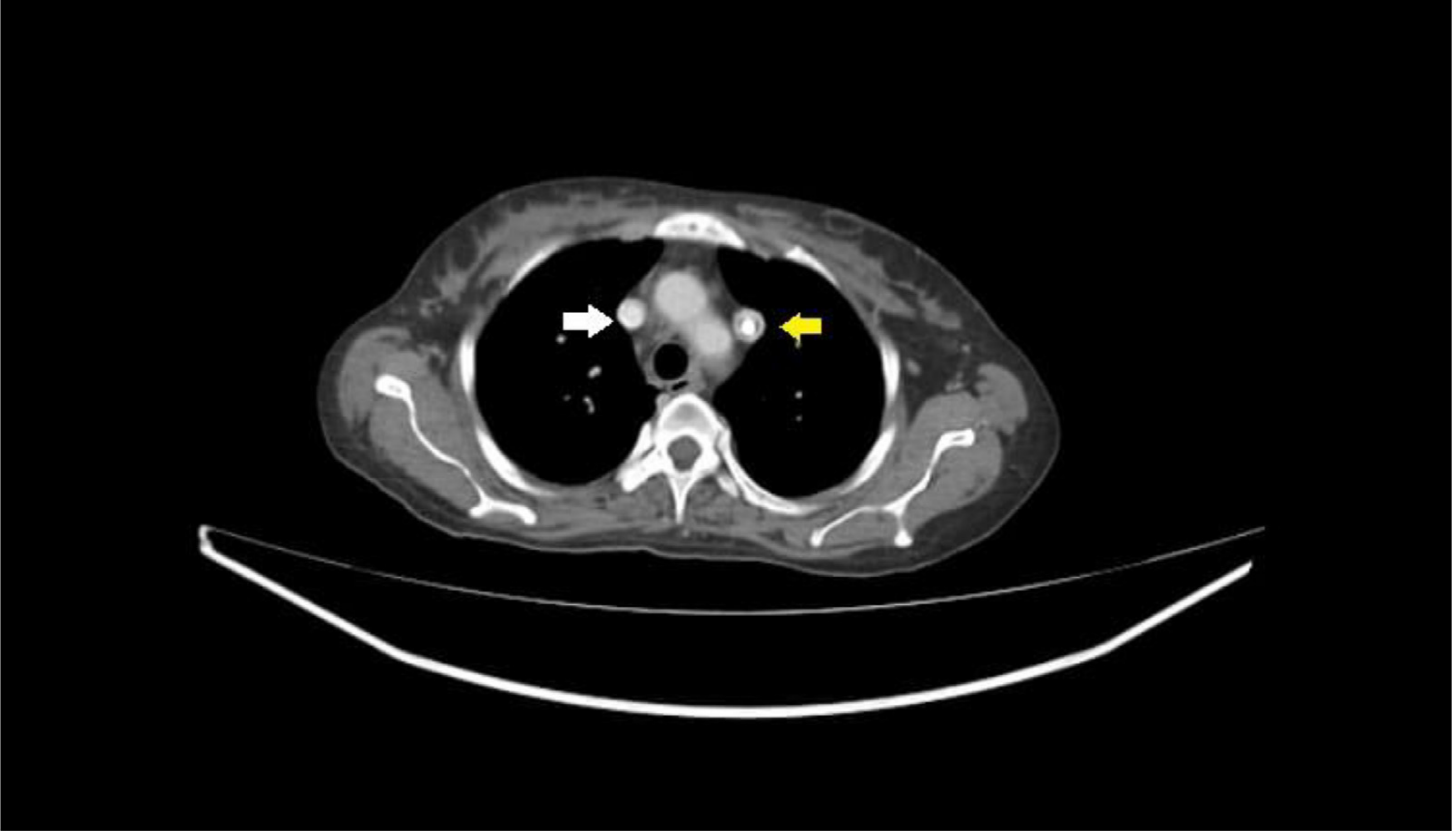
Axial view of CT scan (Yellow arrow pointing at PLSVC, white arrow pointing at right SVC).
